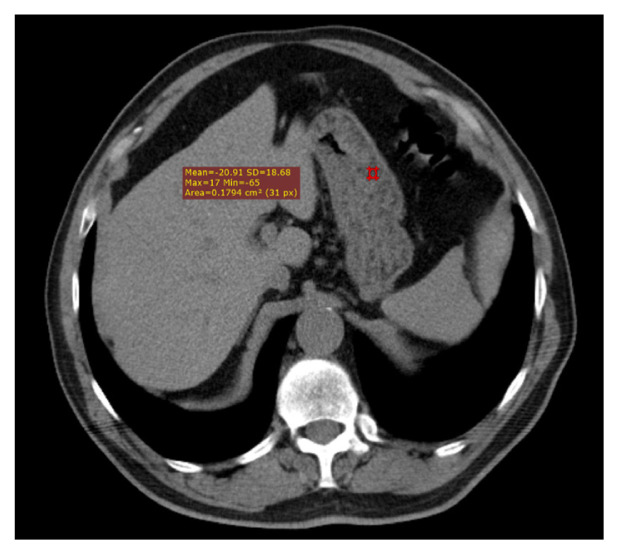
43 year-old man with back pain. Unenhanced thorax CT image represents thin linear fatty infiltration in submucosal layer of gastric wall with attenuation value <−10 HU.
